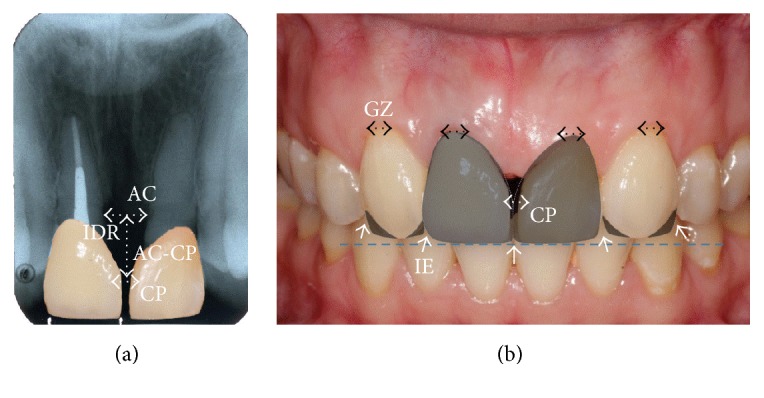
Analysis of “pink” and “white” esthetics and the restorative plan. (a) Radiographic analysis. IDR, the interproximal distance between roots; AC-CP, the distance between the most coronal part of the alveolar bone crest (AC) and the most apical part of the contact point (CP). (b) Clinical photograph analysis. Gingival zenith (GZ) positions, level of upper incisal edge (blue line), incisal edge embrasure (IE), and deficient interdental papilla (black triangle between two teeth) after placement of adhesive restorations are shown. Hyaluronic acid filler injection is planned to fill the deficient interdental papilla.
